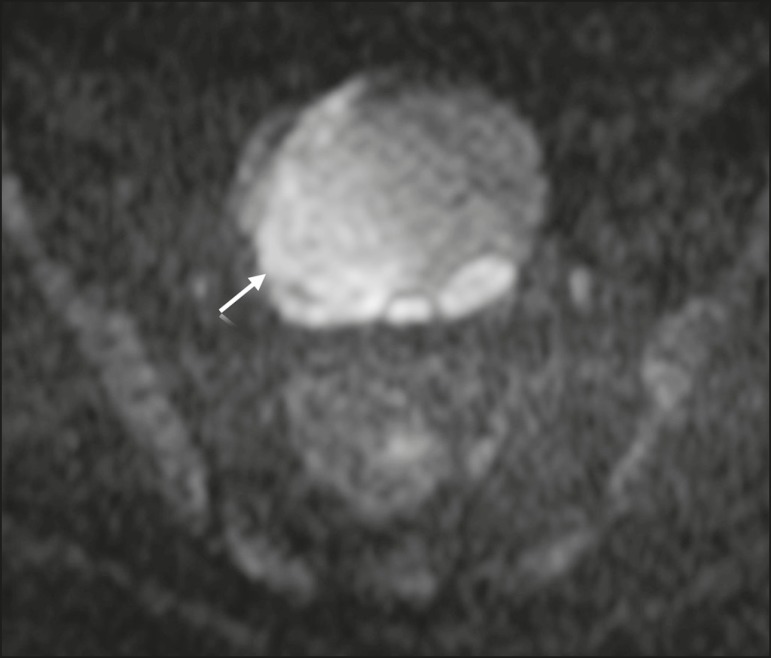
MRI of a female patient with mature cystic teratoma. Axial DWI at b =
